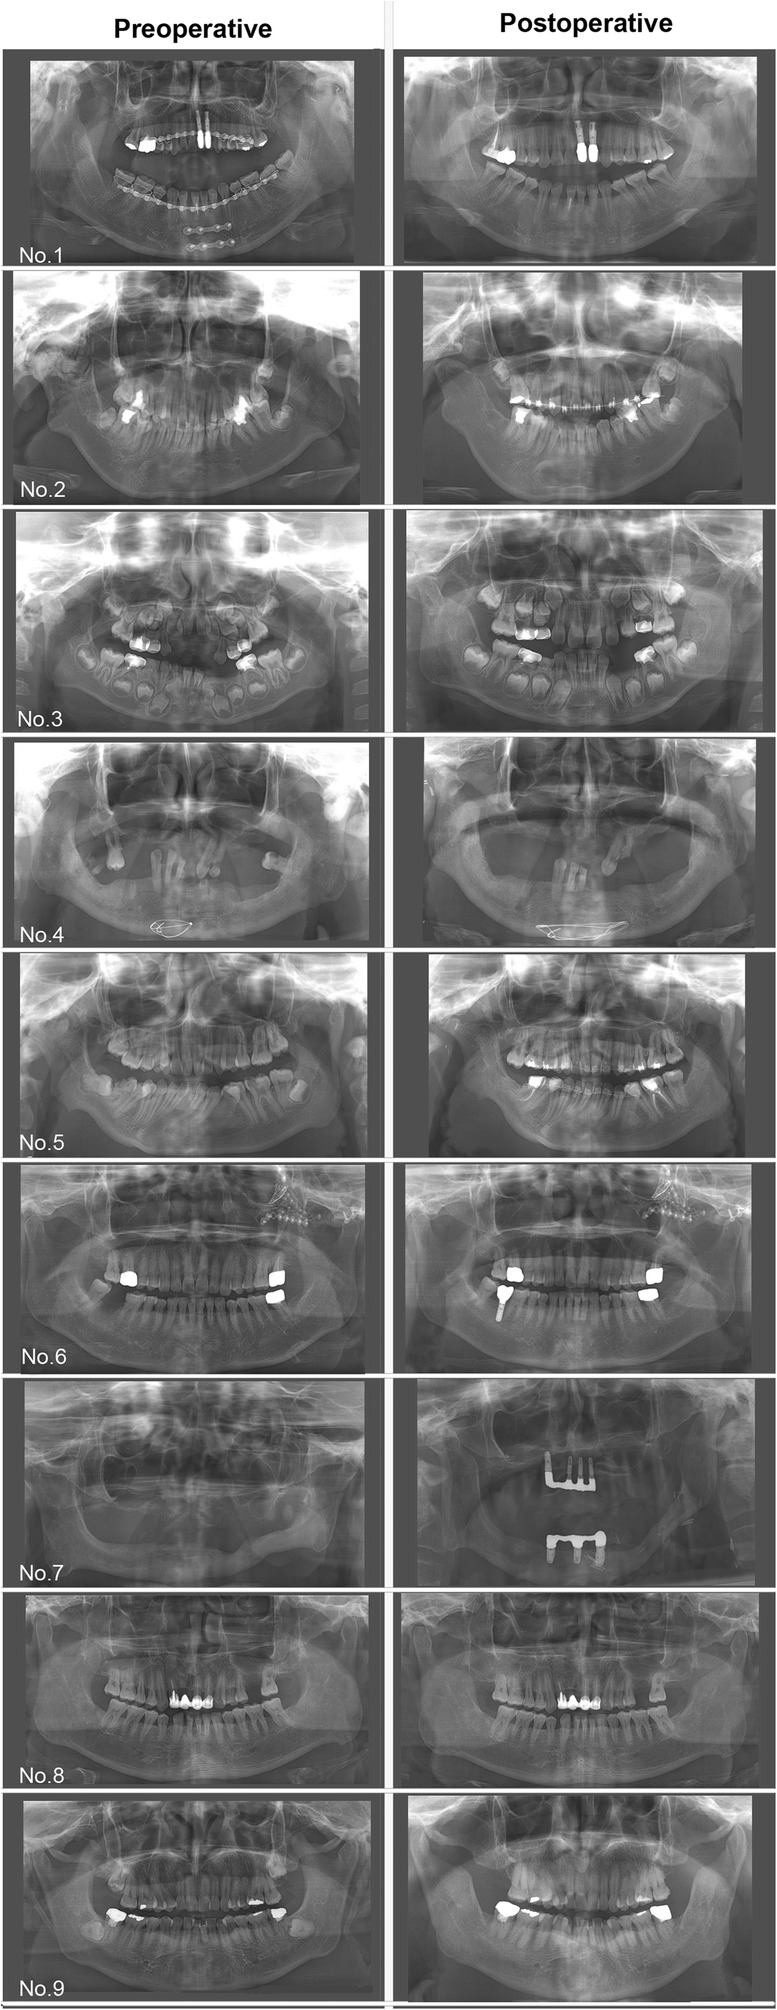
Preoperative and postoperative panoramic radiograms of nine patients according to each sequential number in Table 1. Each postoperative radiogram is taken on the final follow-up time as shown in Table 1
